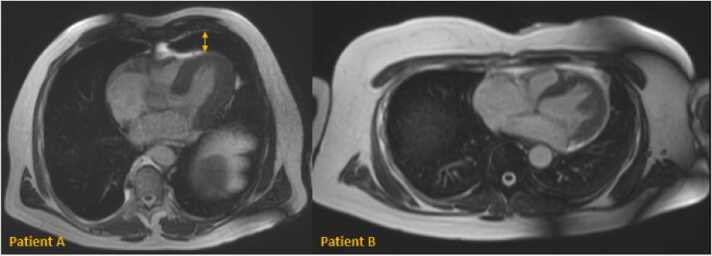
Planning sequences in two different patients (A and B). Patient A had a prominent lingula of the lung (arrow) which led to a poor acoustic window and poor trigger signal and hence to non-diagnostic images of Doppler ultrasound (DUS) gating. Patient B has optimal anatomic conditions (heart directly adjacent to the thoracic wall) for DUS gating which lead to excellent image quality
